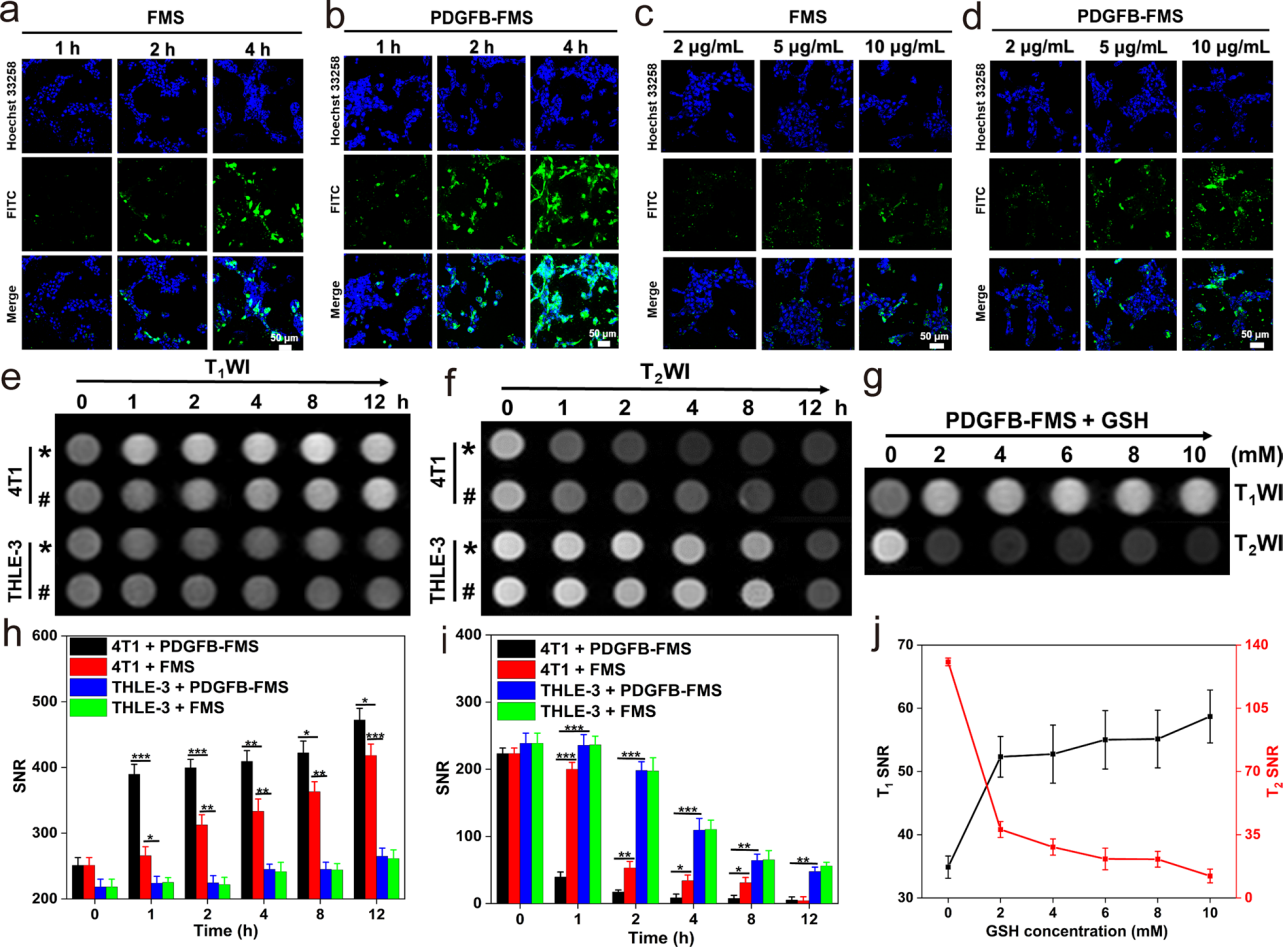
a, b CLSM observation: the internalization process of 4T1 cells treated with FMS and PDGFB-FMS at a certain concentration of 10 μg/mL for 1, 2, and 4 h. c, d CLSM observation: the internalization process of 4T1 cells treated with different concentrations of FMS and PDGFB-FMS for 4 h. e T_1_WI and f T_2_WI of the cells treated with different samples (* and # represent PDGFB-FMS and FMS, respectively) at different time points, and h, i the corresponding T_1_ and T_2_ MRI SNR analysis. g T_1_WI and T_2_WI of 4T1 cells treated with PDGFB-FMS at the presence of different concentrations of GSH, and j the corresponding T_1_ and T_2_ MRI SNR analysis
